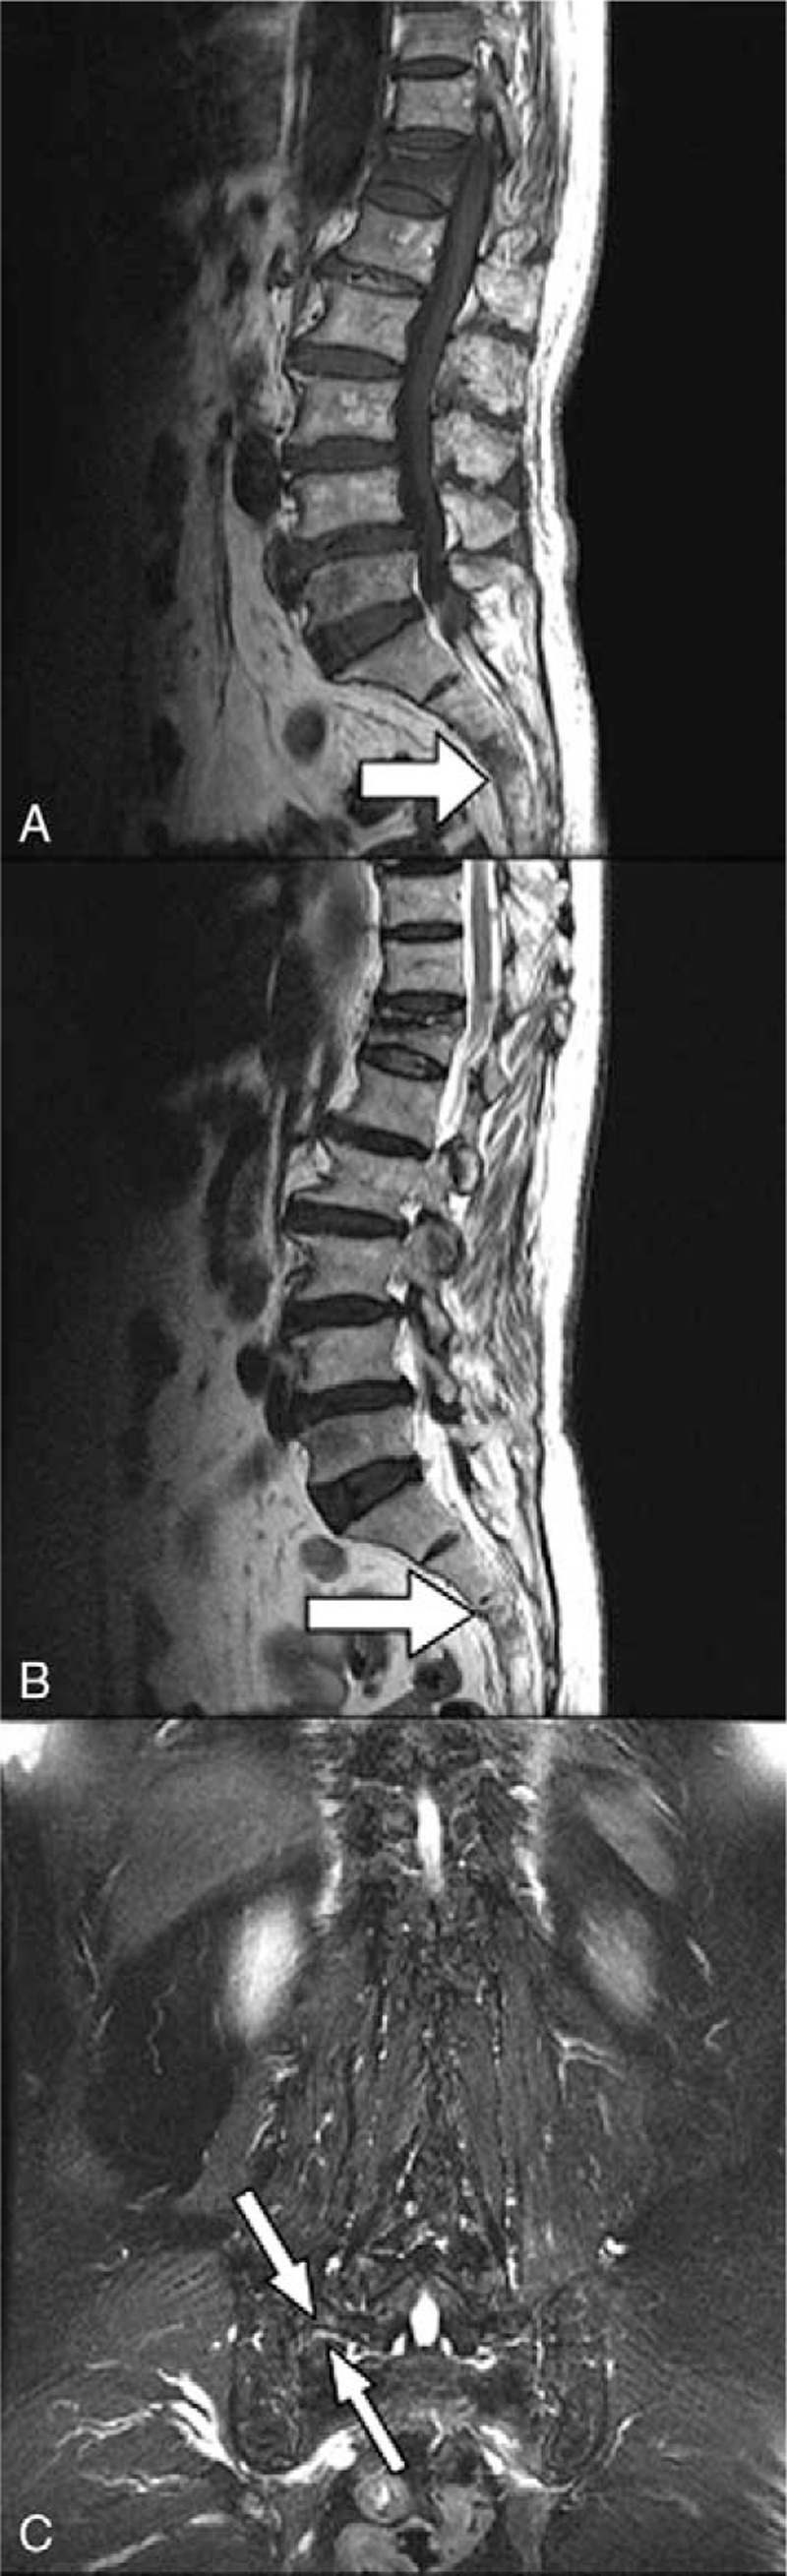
MRI (A, T1-weighted sagittal view; B, T2-weighted sagittal view; and C, T2-weighted coronal view) of a 77-year-old woman with sacral insufficiency fracture. SIF involve S3 body level (white arrow) (B) and transverse alar area (white arrowheads) (C). MRI = magnetic resonance imaging, SIFs = sacral insufficiency fractures.
